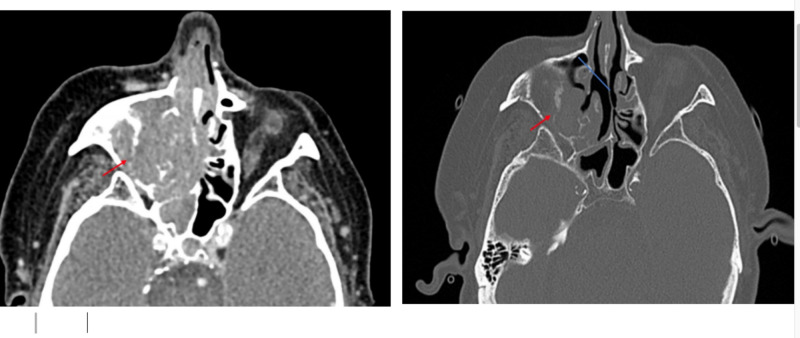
Case one computed tomography Left: Before treatment; red arrow denotes mass; Right: Two months after starting treatment
